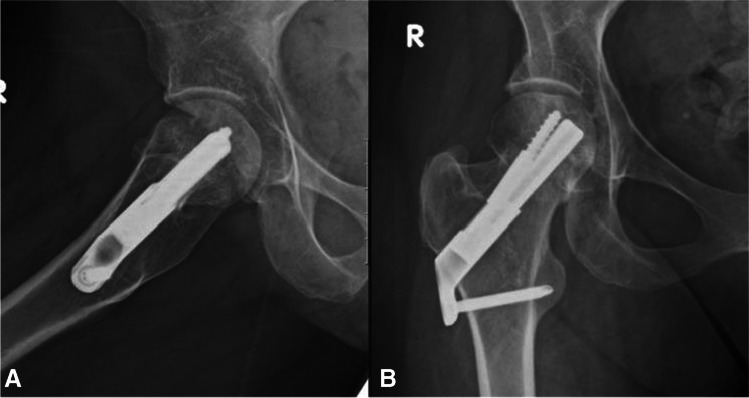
A, B AP and lateral radiographs from last follow-up 27 months after surgical treatment. Returned to her preinjury mobility status
